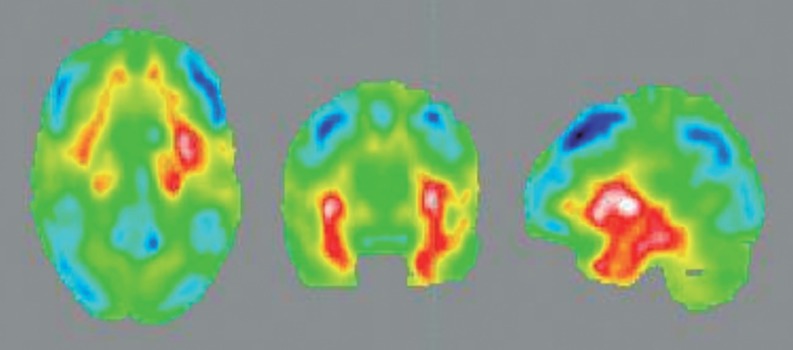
Positron Emission Tomography with fluorodeoxyglucose (PET-FDG) showing increased metabolism in left mesial temporal region and peri-insular cortex (red). Severe and diffuse hypometabolism in frontal, parietal and lateral temporal cortex is also demonstrated (blue).
